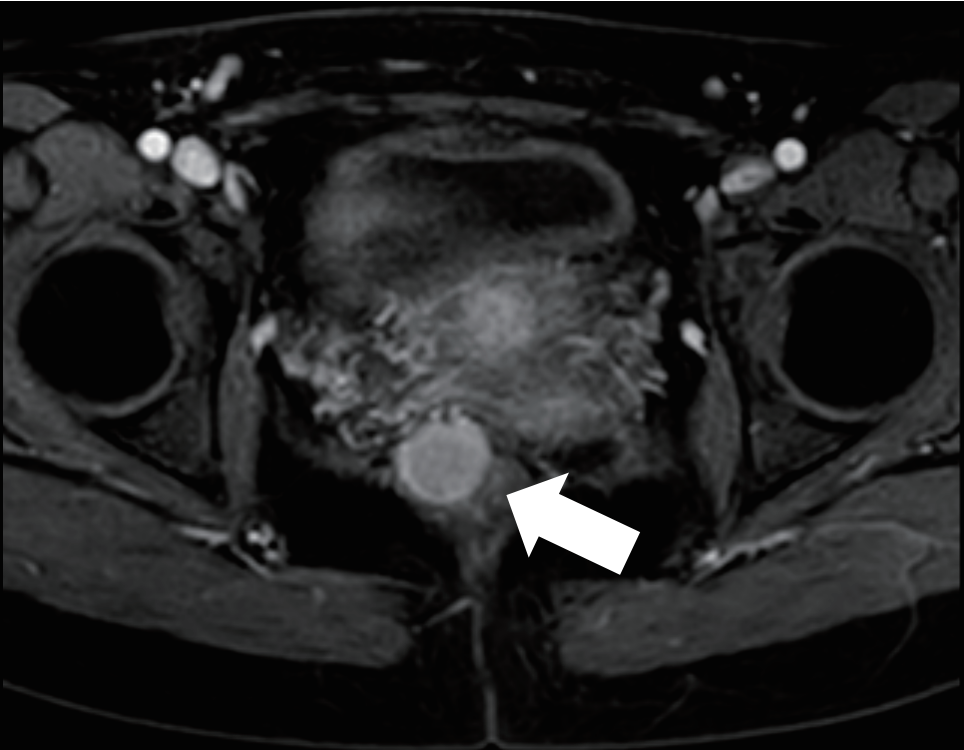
Pelvic MRI findings. The rectal tumor (white arrow) is in the form of a 20-mm submucosal tumor in the lower rectum. MRI, magnetic resonance imaging
